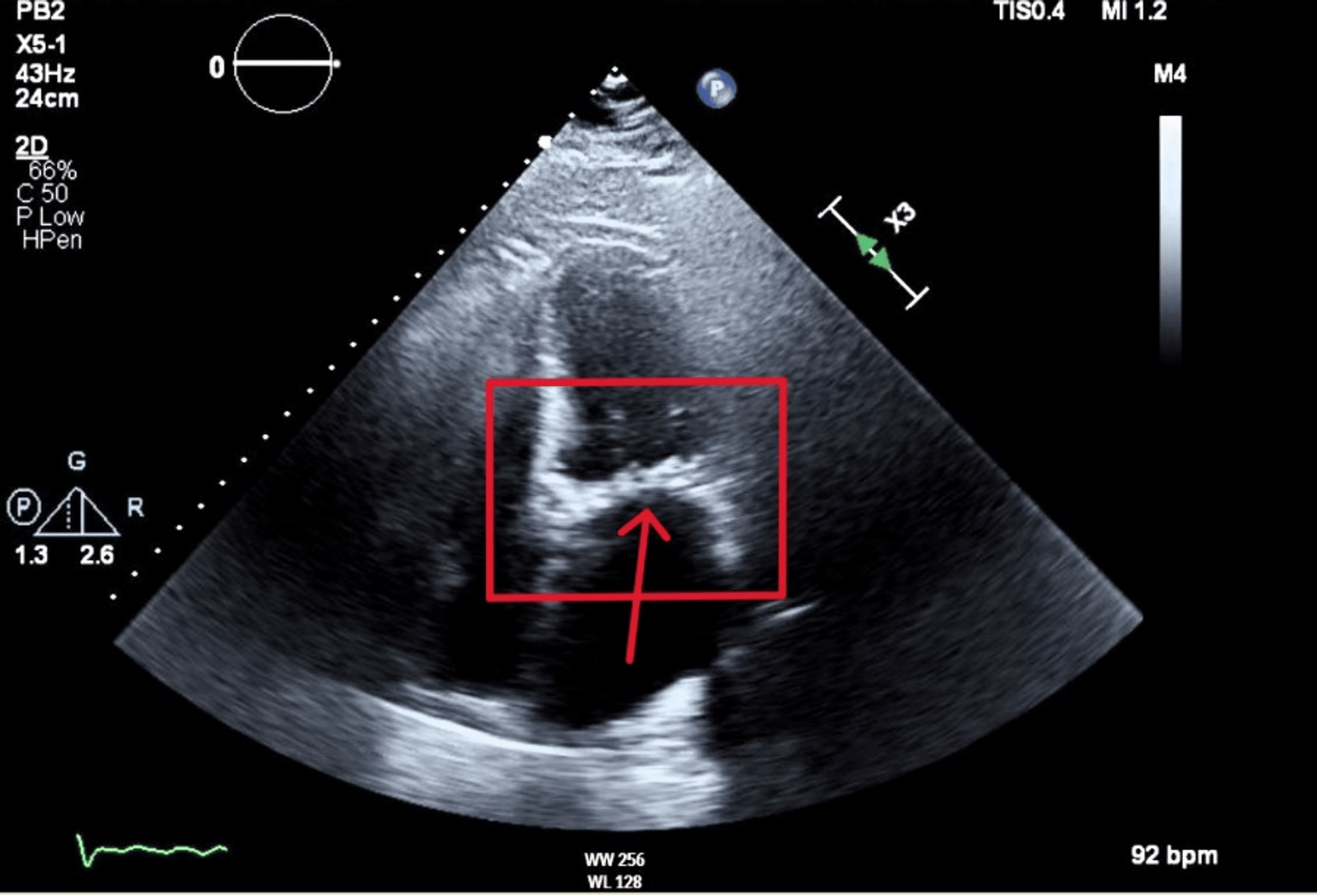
Apical four chamber view of mitral valve stenosis on echocardiogram (red arrow in red box)
